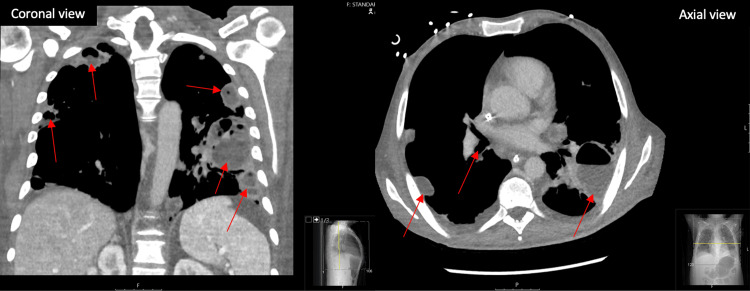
Chest CT with contrast in the coronal and axial view 14 days after admission showing worsening signs of cavitary lesions Red arrows indicate cavitary lesions.
